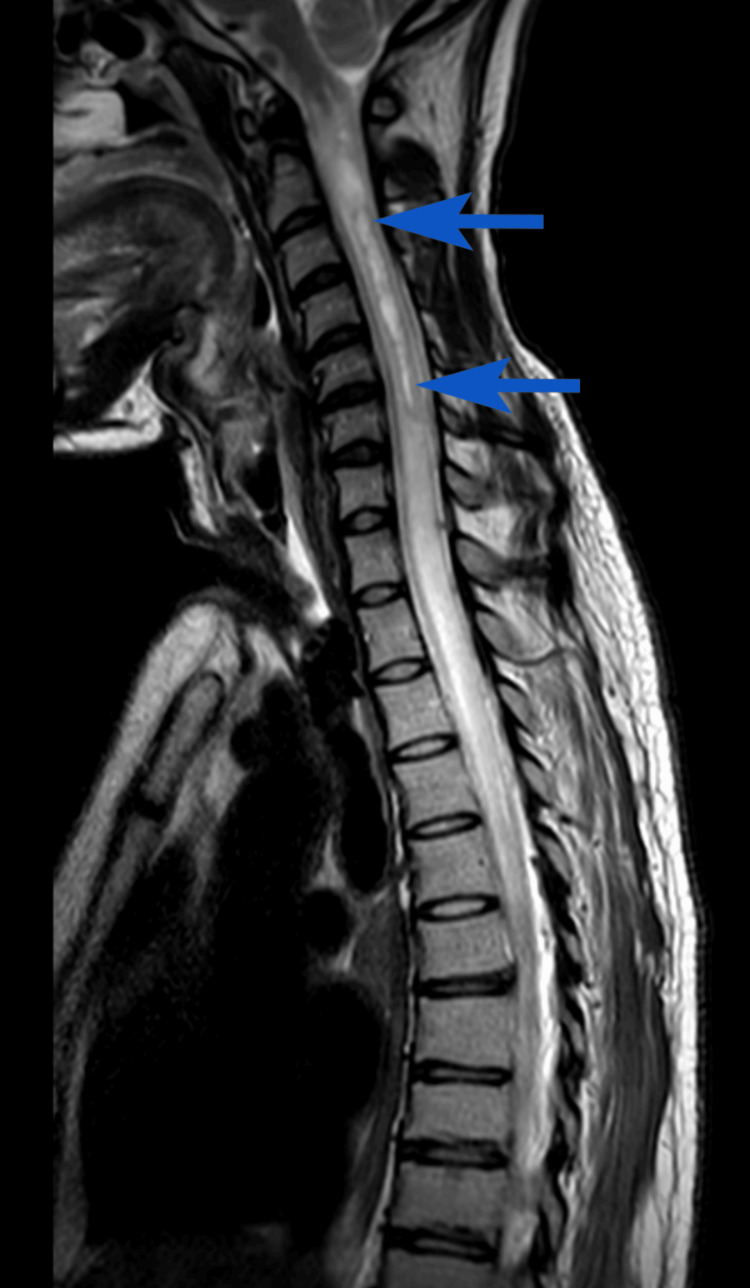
MRI spine sagittal T2-weighted view demonstrating a syrinx extending from C1 to C4. MRI: magnetic resonance imaging, blue arrow: syrinx
